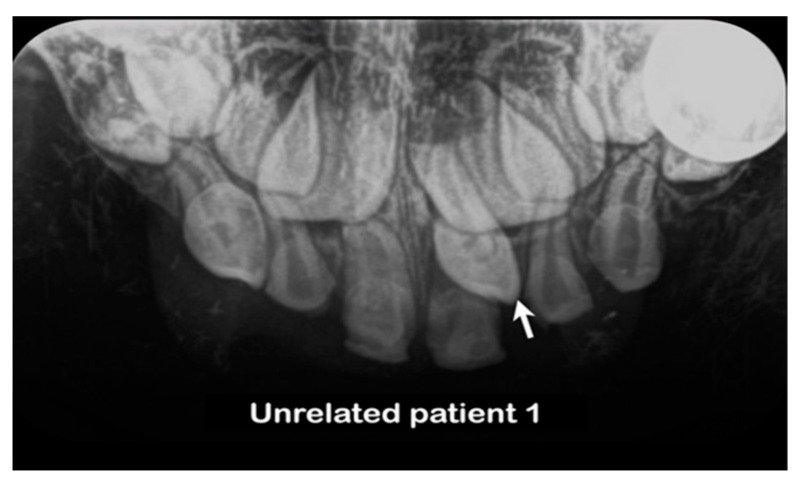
Periapical radiograph of mesiodens of the unrelated patient 1 (arrow).
